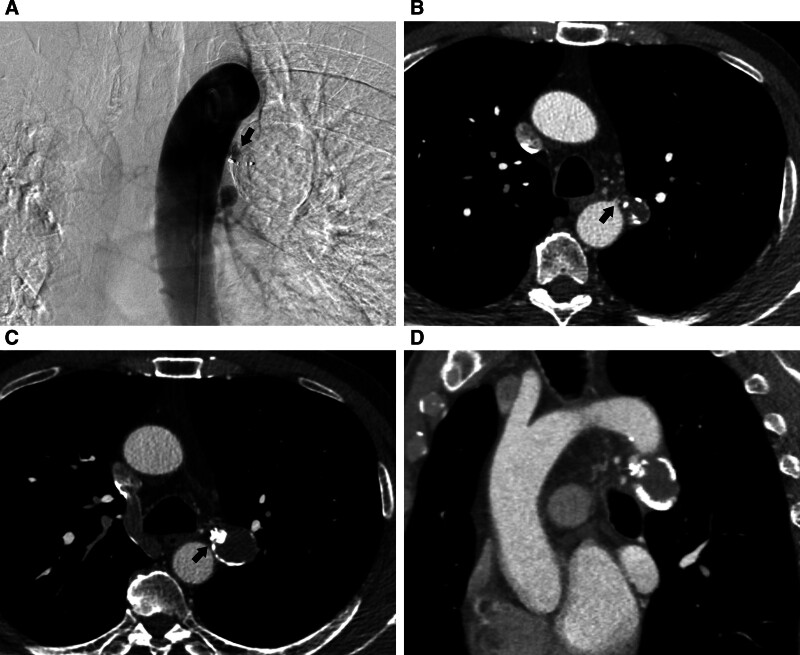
(A) Aortic angiography revealed no signs of aneurysm recurrence 1-month after embolization; (B, C) the CTA performed 6 months after embolization showed a reduction in the size of the aneurysm neck (black arrow) and no signs of aneurysm recurrence (black arrow); and he oblique coronal chest CTA also revealed no signs of aneurysm recurrence. CTA = computed tomography angiography.
